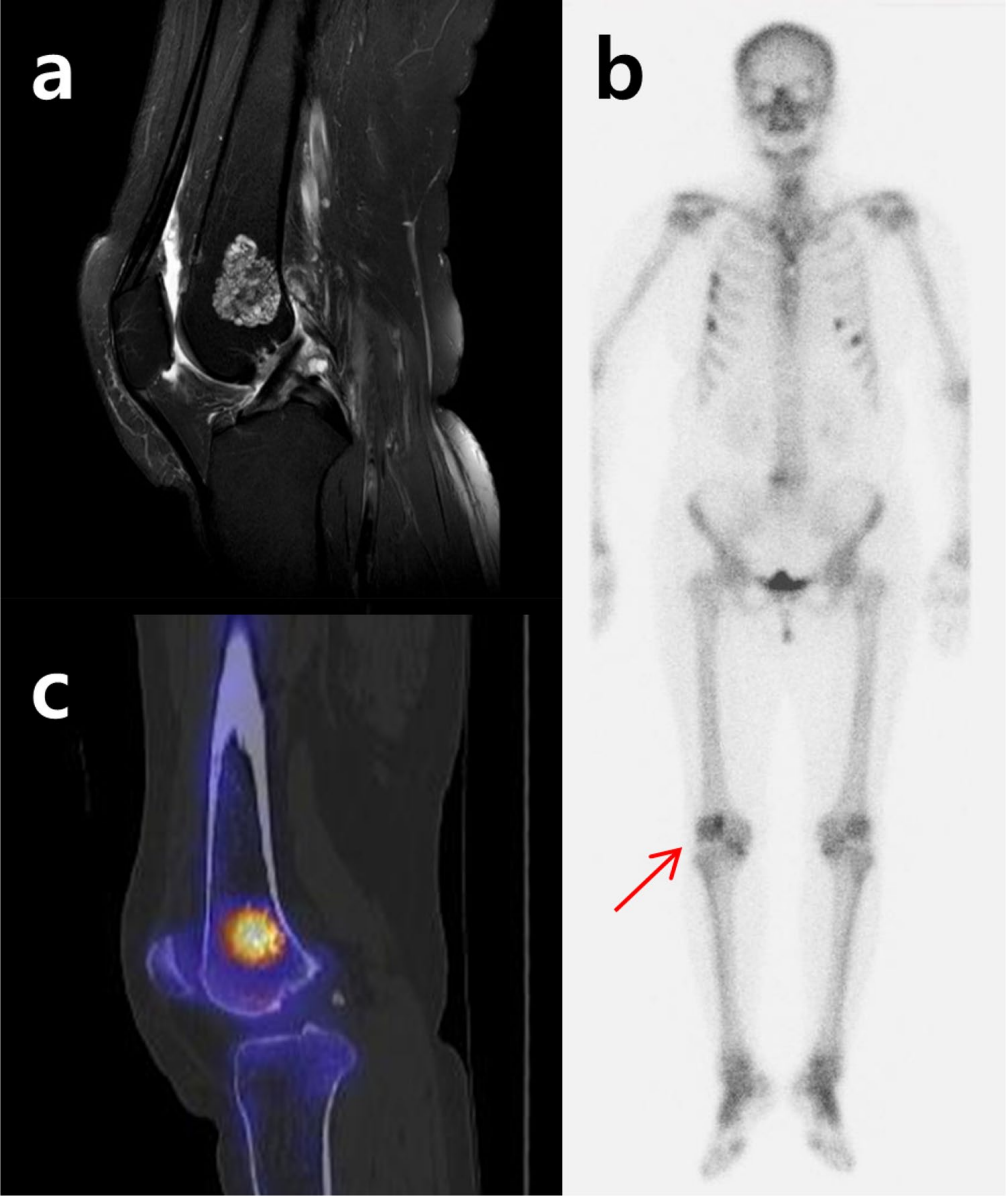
A 76-year-old female patient who was diagnosed with enchondroma 9 years ago. (a) Follow-up MRI shows no interval change. On fat-suppressed contrast-enhanced T1-weighted images, about 3.2 cm sized mass with nodular and septal enhancement in distal metaphysis of femur is shown. (b) Whole body bone scintigraphy demonstrates focal radionuclide uptake on right distal femur (arrow) which has higher uptake than anterior iliac crest. (c) On the SPECT/CT images, the SUVmax of the lesion was calculated to be 14.7.
